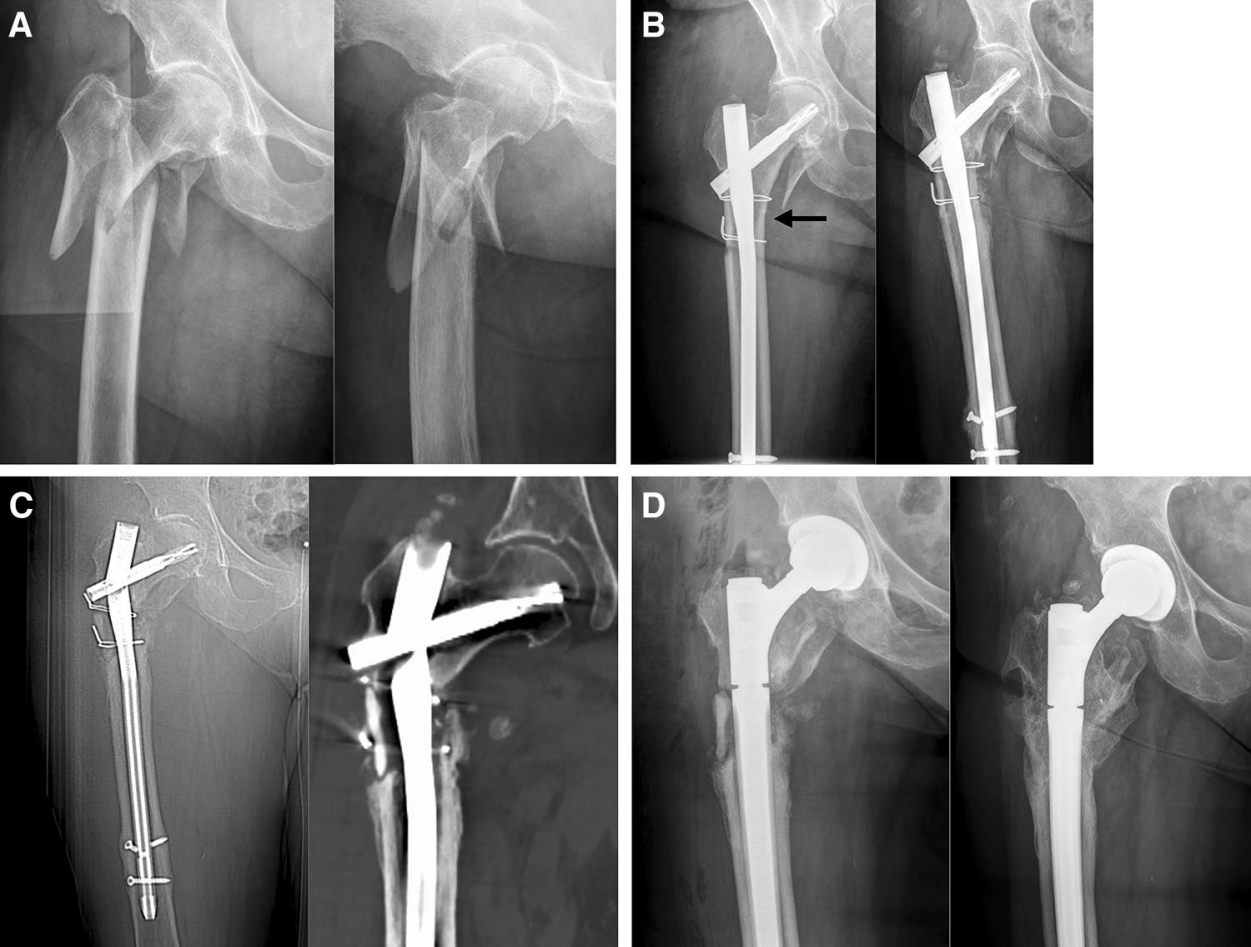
Eighty three-year-old female after a simple fall at home. a Radiographs obtained at admission showing a Seinsheimer Type V fracture. b Postoperative radiograph (left) after open reduction, cerclage wiring and intramedullary nailing: restoration of the subtrochanteric medial cortical support (black arrow) and no varus malalignment. The lesser trochanter fragment was not reduced. Scheduled radiograph after 12 weeks: no loss of reduction, timely callus formation, but breakage of the static locking bolt and autodynamisation of the nail. c Unscheduled radiograph (left) and CT scan (right) after 10 months due to suddenly increasing pain: nail breakage and no fracture healing. d Postoperative X-ray (left) after conversion to total hip arthroplasty using a modular revision stem anchored in the diaphyseal isthmus. The nonunion was not addressed surgically. Scheduled radiograph (right) 12 months after revision surgery: no component loosening and fracture healing
